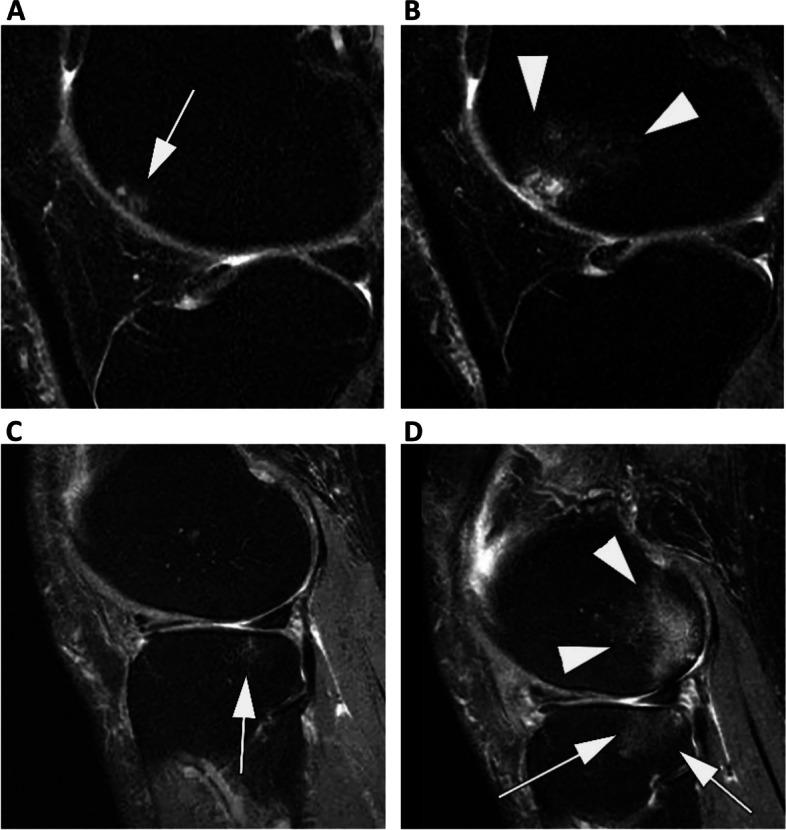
Examples of change in bone marrow lesions (BML). One of the outcome measures was change in maximum size of BML per knee. A Baseline sagittal intermediate-weighted fat suppressed MRI shows a small grade 1 BML at the anterior lateral femur (arrow). B 24-month follow-up MRI shows increase in size of BML to grade 2 (arrowheads). C Another example shows a small grade 1 BML at the posterior lateral tibia at baseline (arrow). D 24-month follow-up MRI shows marked increase in tibial BML now comprising the central and posterior tibial subregions (arrows). In addition, there is a new grade 3 BML at the lateral posterior femur (arrowheads) that also involves the central lateral femur (grade 1). This knee shows an increase of number of subregions affected by any BML from 1 subregion at baseline to 4 subregions at 24 months follow-up. Increase in number of subregions affected per knee was the second outcome measure in this study
